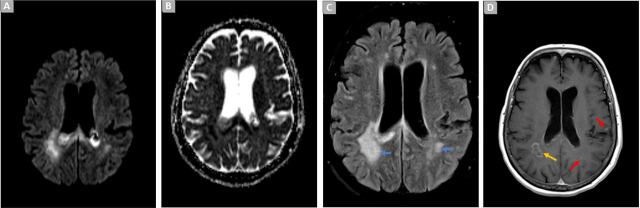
Brain MRI. Axial diffusion weighted image (A), ADC map (B), T2/FLAIR (fluid-attenuation-inversion-recovery) (C) and T1 SE post contrast (D), showing increased signal in T2iFLAIR image at the adjacent bilateral periventricular parenchyma [C, blue arrows], with restricted diffusion [A-B] and anomalous contrast enhancement [D], exhibiting signs of loculation in the right parietal white matter [D, yellow arrow] and some focal contralateral nodules [I), red arrows].
